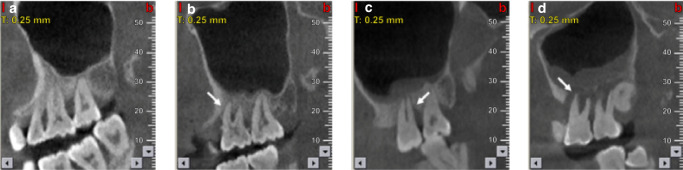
Cone beam computed tomography (CBCT) images of maxillary sinus mucosa.^25^
